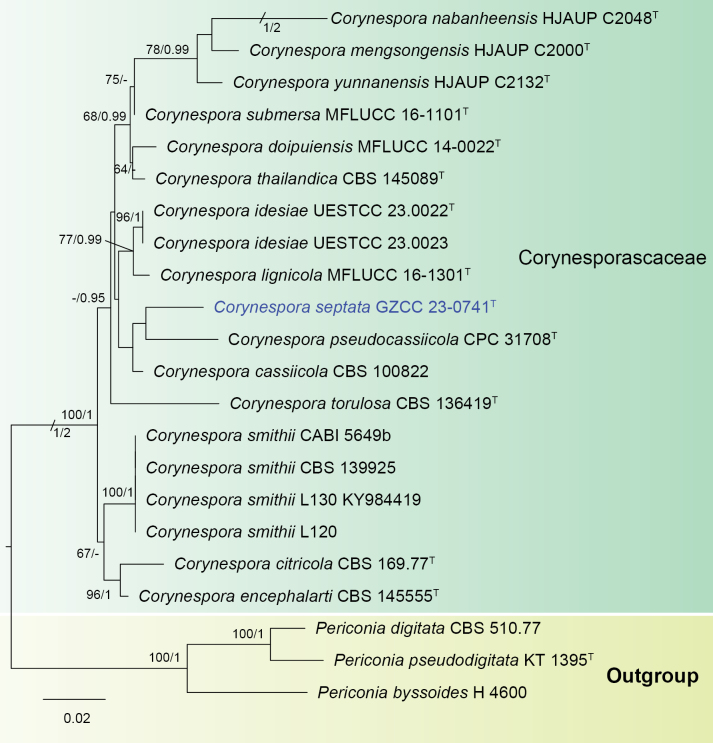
Phylogram generated from maximum likelihood analysis based on combined LSU and ITS sequence data. Twenty-two taxa were included in the combined analyses, which comprised 1393 characters (LSU = 844 bp and ITS = 549 bp) after alignment. The best scoring RAxML tree with a final likelihood value of -4677.993509 is presented. The matrix had 336 distinct alignment patterns, with 9.65% of undetermined characters or gaps. Estimated base frequencies were as follows: A = 0.243499, C = 0.247400, G = 0.289966, T = 0.219136; substitution rates: AC = 3.067252, AG = 2.397685, AT = 1.551875, CG = 1.069828, CT = 6.624253, GT = 1.000000; gamma distribution shape parameter *α* = 0.240919. Bootstrap support values for ML equal to or greater than 60% and prior probabilities (PPs) equal to or greater than 0.95 are given above the nodes as ML/PP. The tree was rooted to *Periconiabyssoides* (H 4600), *P.digitata* (CBS 510.77) and *P.pseudodigitata* (KT 1395). The strain numbers are noted after the species names with ex-type strains indicated by ^T^. The newly generated sequences are indicated in blue.
